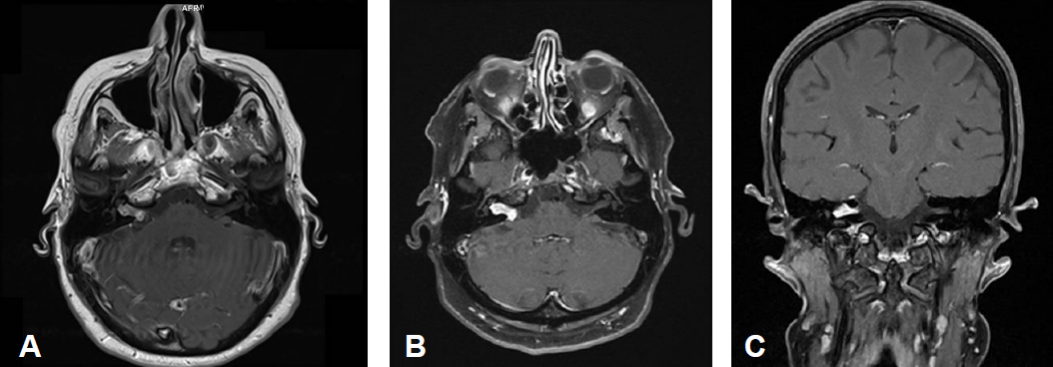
MRI, ; A axial, initial findings; T1 FS + CM, control after 4 months without therapy (B axial, C coronal).
